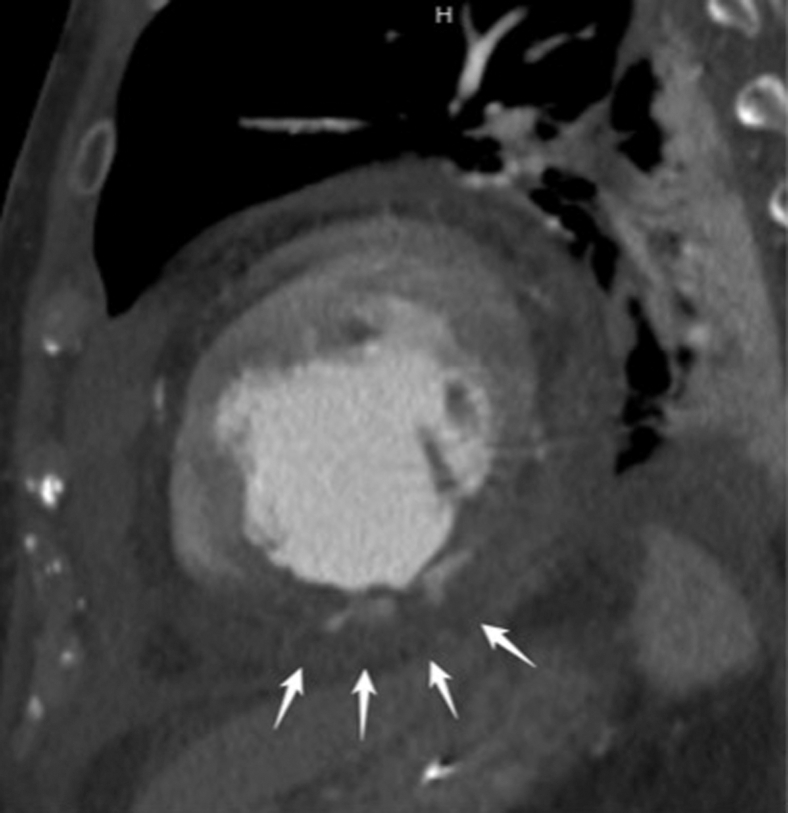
Recurrent hemorrhagic chest-tube output prompted contrast-enhanced computed tomography revealing active contrast extravasation from the posterior LV wall (*white arrows*).
